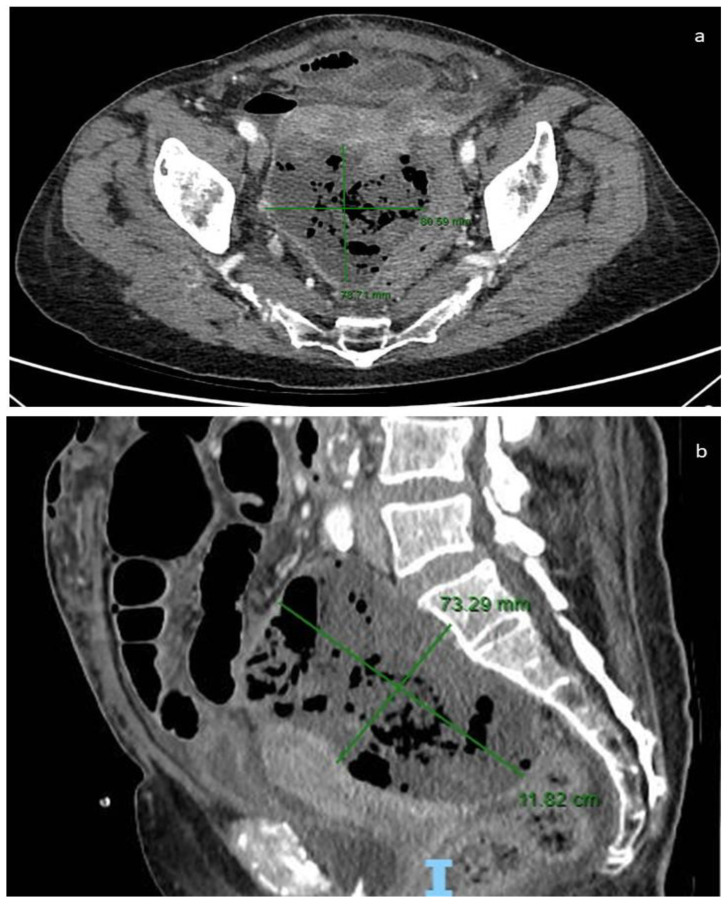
Our case: CT image with measurements: axial plane (a) and sagittal plane (b).
